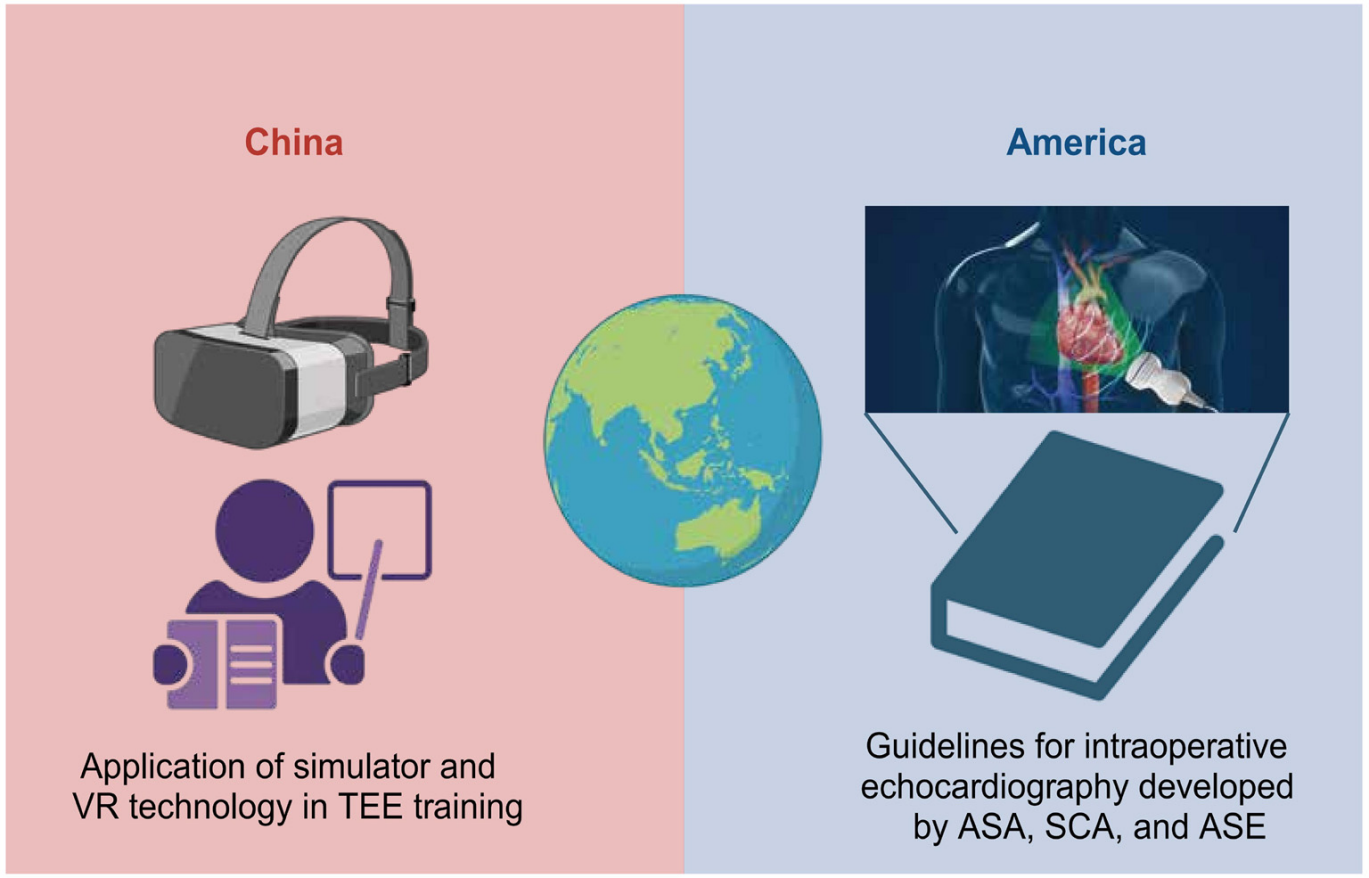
A global perspective on the standardization of TEE education and practice. TEE: Transesophageal echocardiography; VR: Virtual reality; ASA: The American Society of Anesthesiologists; SCA: Society of Cardiovascular Anesthesiologists; ASE: The American Society of Echocardiography.
